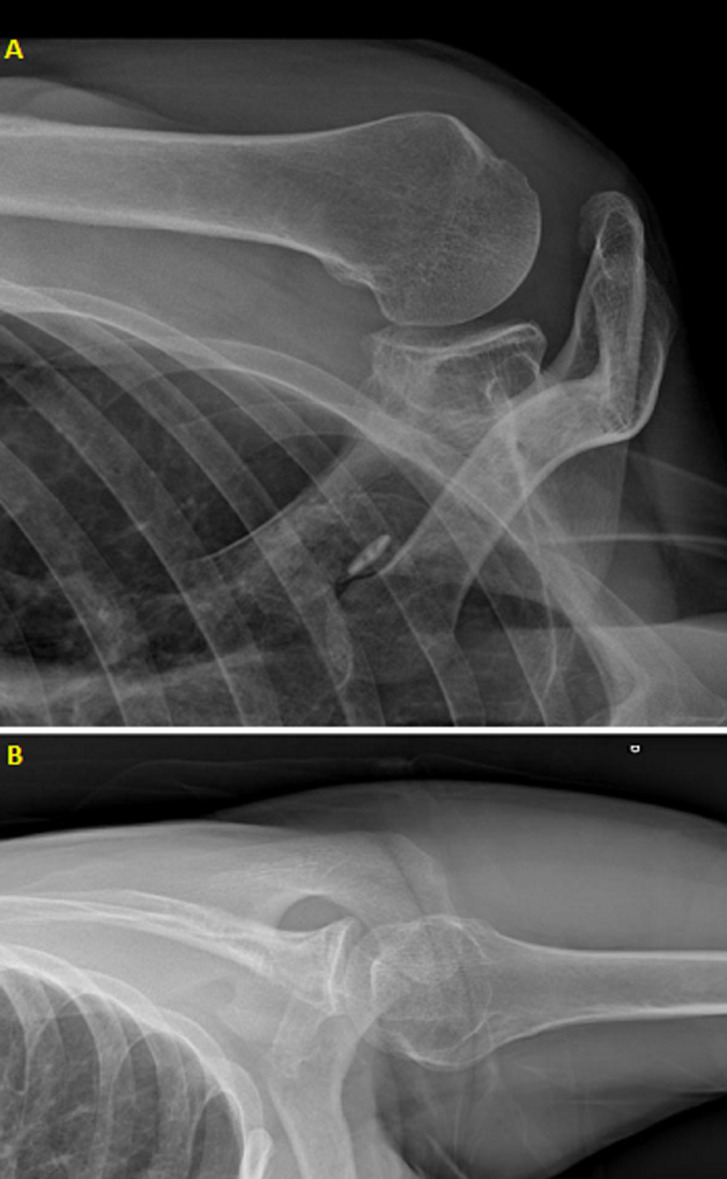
(A,B) X-rays at 13 months after surgery (front and axillary side)
